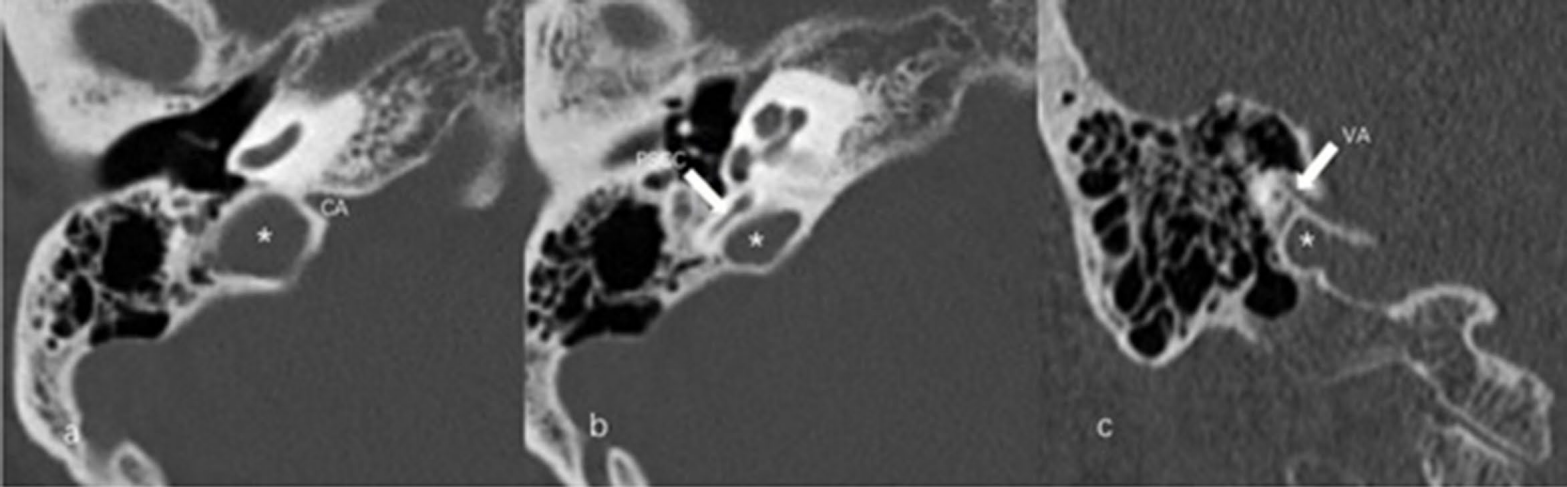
Inner ear adjacent jugular bulb (IAJB). (a) Jugular bulb (JB) (asterisk) is adjacent to the cochlear aqueduct (CA) on the axial image of temporal bone high-resolution computed tomography (HRCT). (b) JB (asterisk) is adjacent to the posterior semicircular canal (arrow) on the axial image of temporal bone HRCT. (c) JB (asterisk) is adjacent to the vestibular aqueduct (arrow) on the coronal image of temporal bone HRCT.
